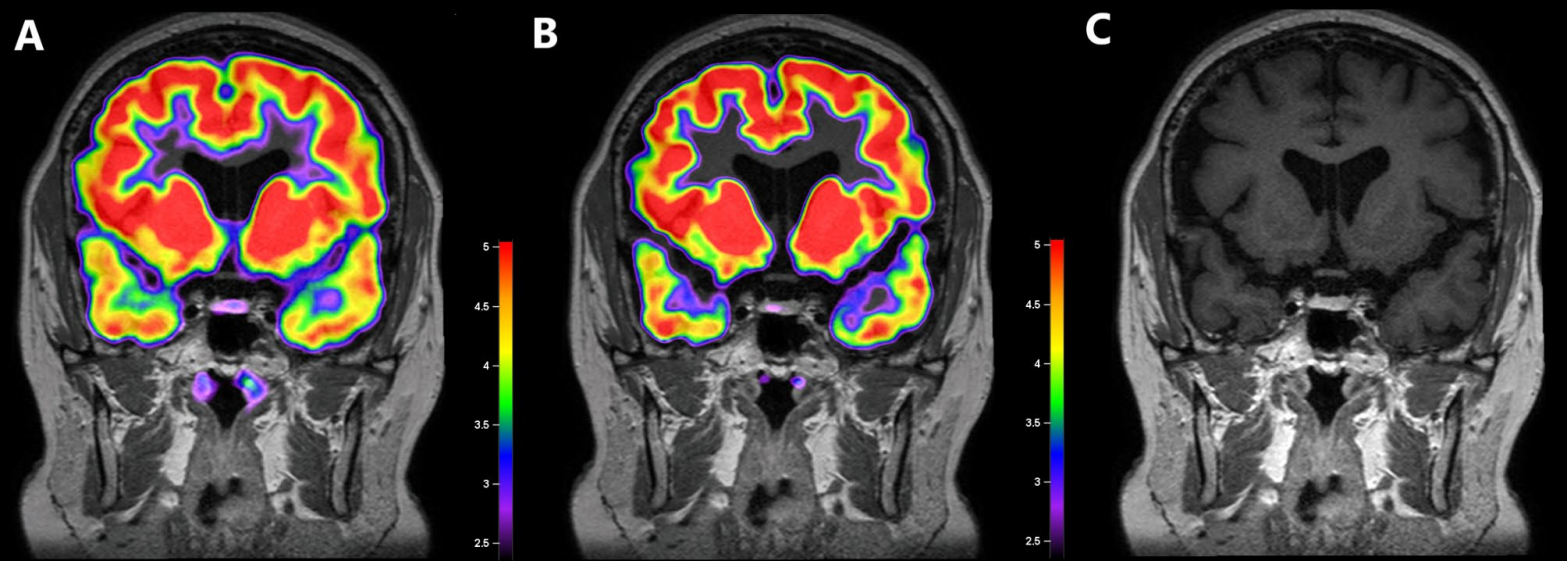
Images of a corticotroph with negative MRI but positive^18^ F-FDG PET/CT after DEX suppression An MRI-negative adenoma was detected on ^18^F-FDG PET/CT at baseline and after DEX suppression. In this patient, the pituitary adenoma was visible on PET scans at baseline (B) and after DEX suppression (C) at the same location, as confirmed by the surgeon A. Co-registered baseline ^18^F-FDG PET/CT and MRI images. Diffuse ^18^F-FDG uptake is detected in the pituitary fossa with an SUV_mean_ of 0.86 and SUV_max_ of 1.03, but there was failure to localize the adenoma on baseline ^18^F-FDG PET/CT. B. Co-registered ^18^F-FDG PET/CT and MRI images after DEX suppression. ^18^F-FDG uptake is not suppressed in the right side of the pituitary gland with an SUV_mean_ of 1.03 and SUV_max_ of 1.06. ^18^F-FDG PET/CT after DEX suppression was successful in localizing the right-sided corticotrophic adenoma C. MRI image. There is no suspicious lesion in the pituitary gland ACTH, adrenocorticotropic hormone; MRI, magnetic resonance imaging; ^18^F-FDG, ^18^ F-fluorodeoxyglucose; PET/CT, positron emission tomography/computed tomography; DEX, dexamethasone; SUV_mean_, mean standardized uptake value; SUV_max_, maximum standardized uptake value
